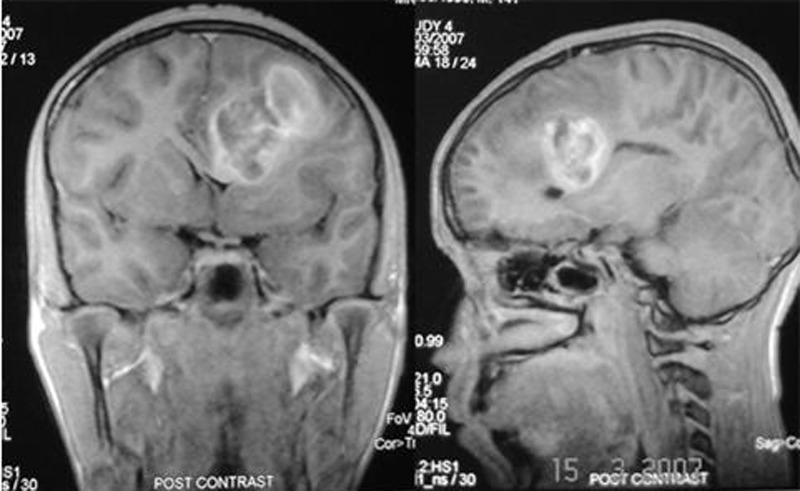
Figure 2: Contrast MRI coronal and sagittal sections showing more details of the lesion; note the mass effect and distortion of corpus callosum.
